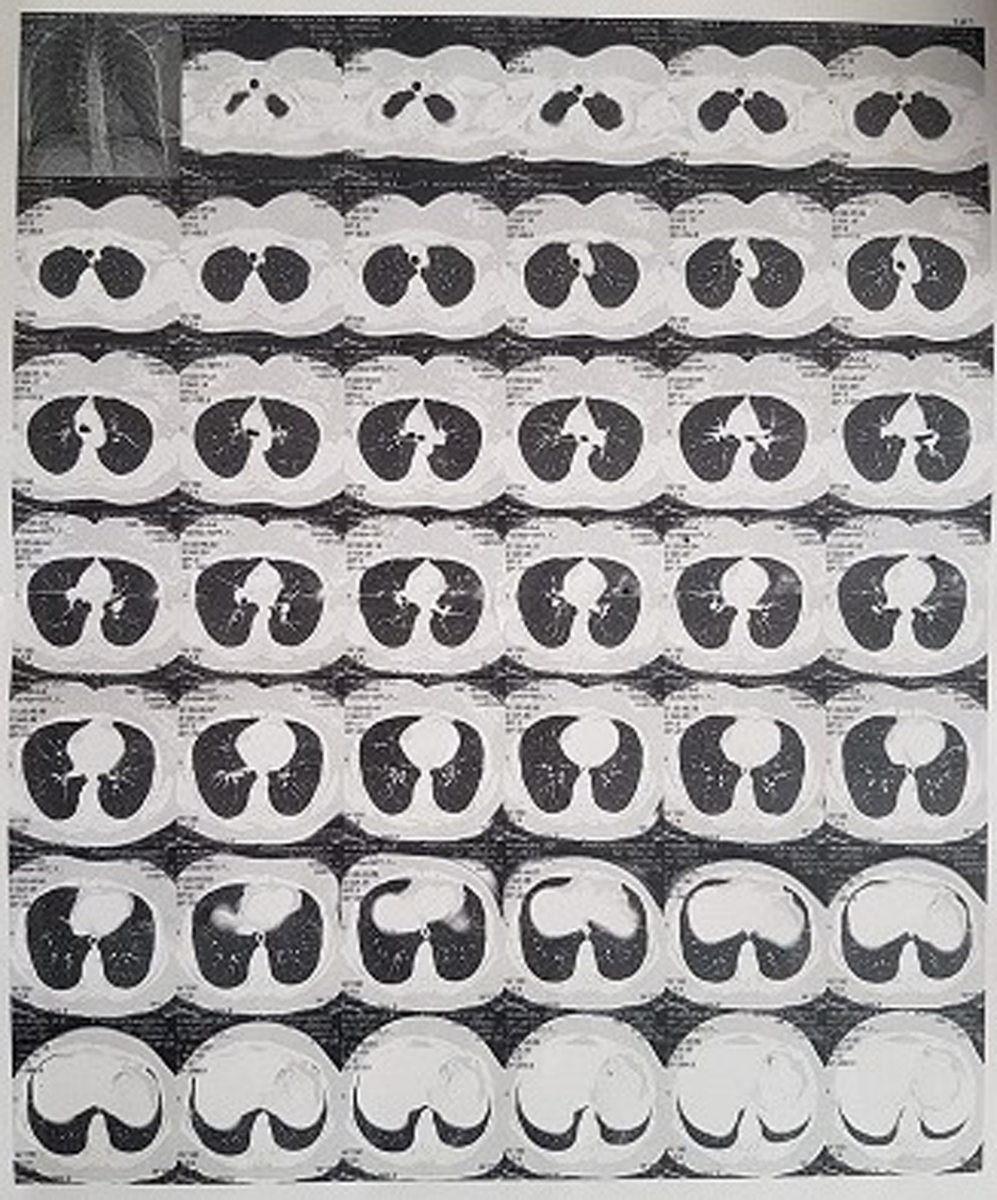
Computed tomography (CT) scan in COVID‐19 patient with unilateral lung involvement.
